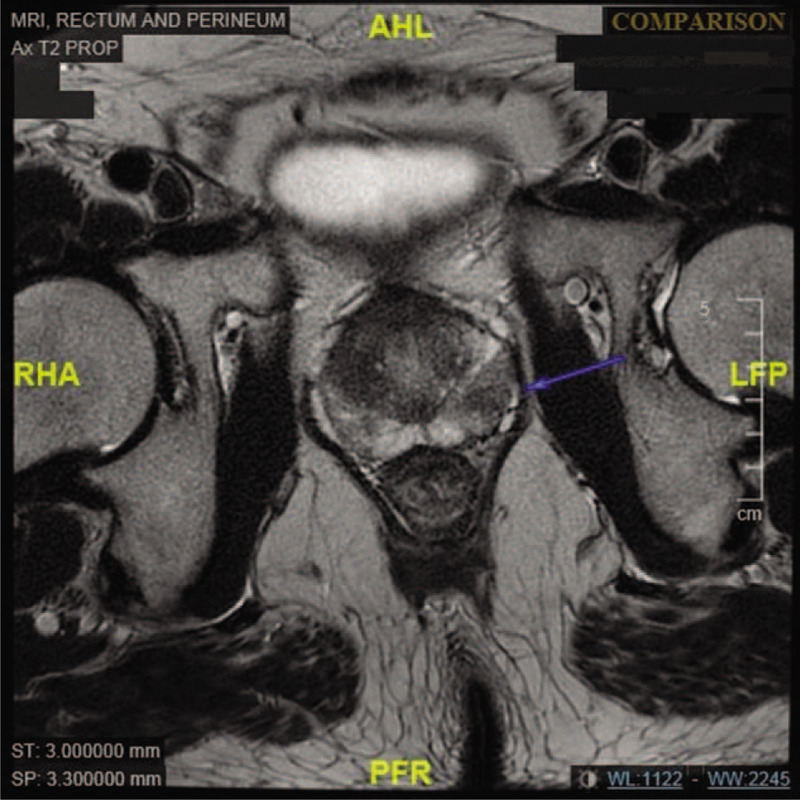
MRI showing prostate adenocarcinoma. Blue arrow indicating the prostate adenocarcinoma.
